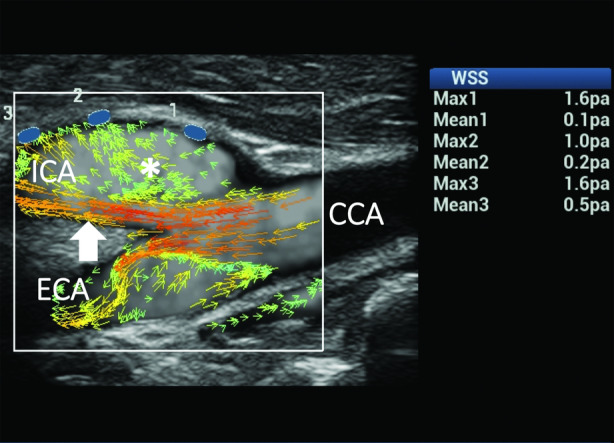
Complex flow in the CB. Ultrasound vector flow imaging shows changes of direction and velocity of the flow layers due to vessel dilatation, resulting in the development of complex flow in both the internal carotid artery (ICA) and external carotid artery (ECA). High‐velocity red vectors flowing near the flow divider in the ICA (arrow) and a wide area of multidirectional low‐velocity green vectors (asterisk) are shown in the ICA sinus. Wall shear stress measurements on the outer wall (blue dots) of the ICA gave normal maximum values (1.0–1.6 Pa) in all of the samples and abnormal mean values (0.1–0.2 Pa) in 2 of the 3 sample points. CCA indicates common carotid artery.
